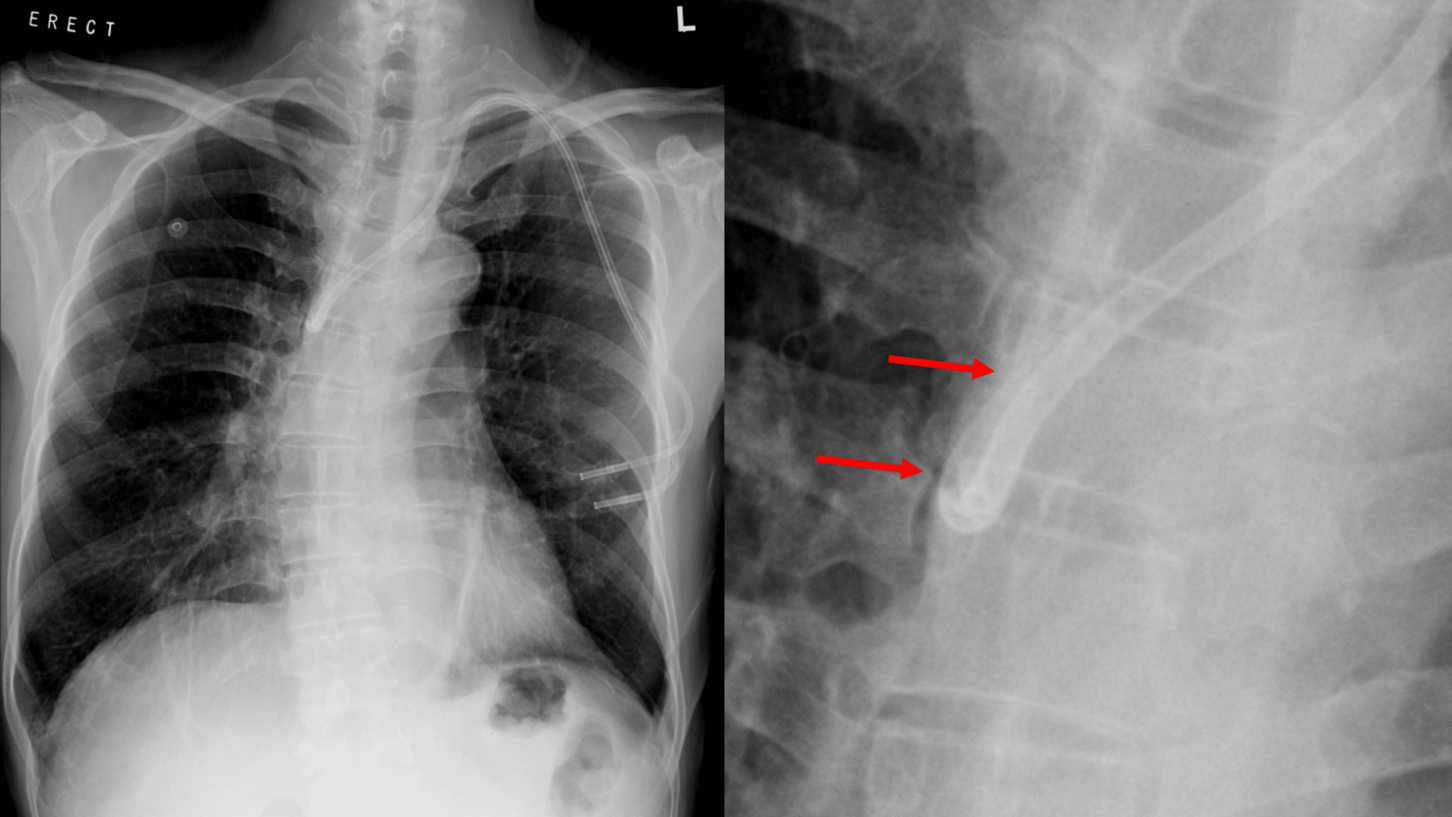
CXR (right) and zoomed in at the catheter tip (left) A left jugular tunneled split-stream CVC is noted. Both tips of the catheter (arrows) are superimposed at the right tracheobronchial angle, with an abnormal fishhook appearance. These findings are highly suggestive of catheter tip malposition into the azygos vein. CVC: malposition of central venous catheter, CXR: chest radiograph
